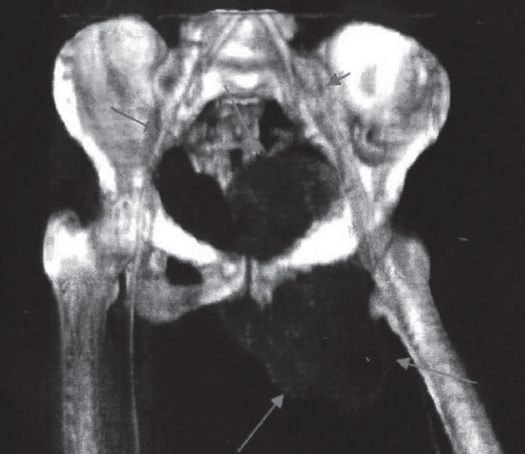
Color volume rendered 3D CT of a left iliac GCT. Note the spatial depiction of the tumor with nearby common iliac vessels
